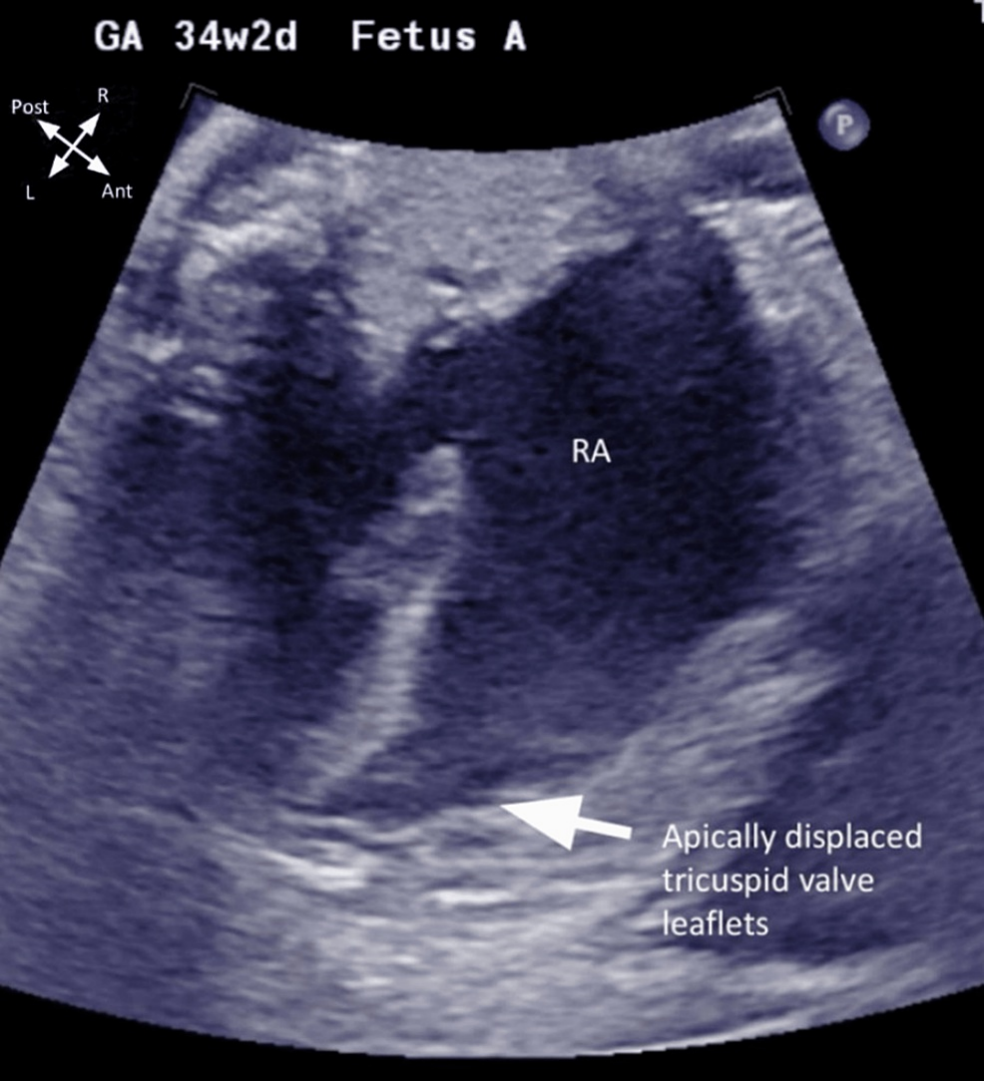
Fetal echocardiogram showing apical four-chamber view of Twin A with Ebstein's anomaly RA, right atrium
